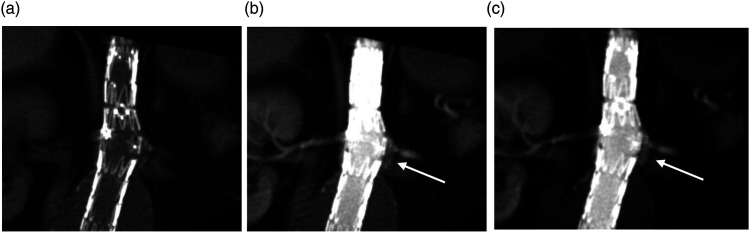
4D CT with unenhanced (a) and early contrast enhanced phases (b and c) demonstrating early contrast enhancement below the left renal stent consistent with a Type 3c leak (white arrows).
